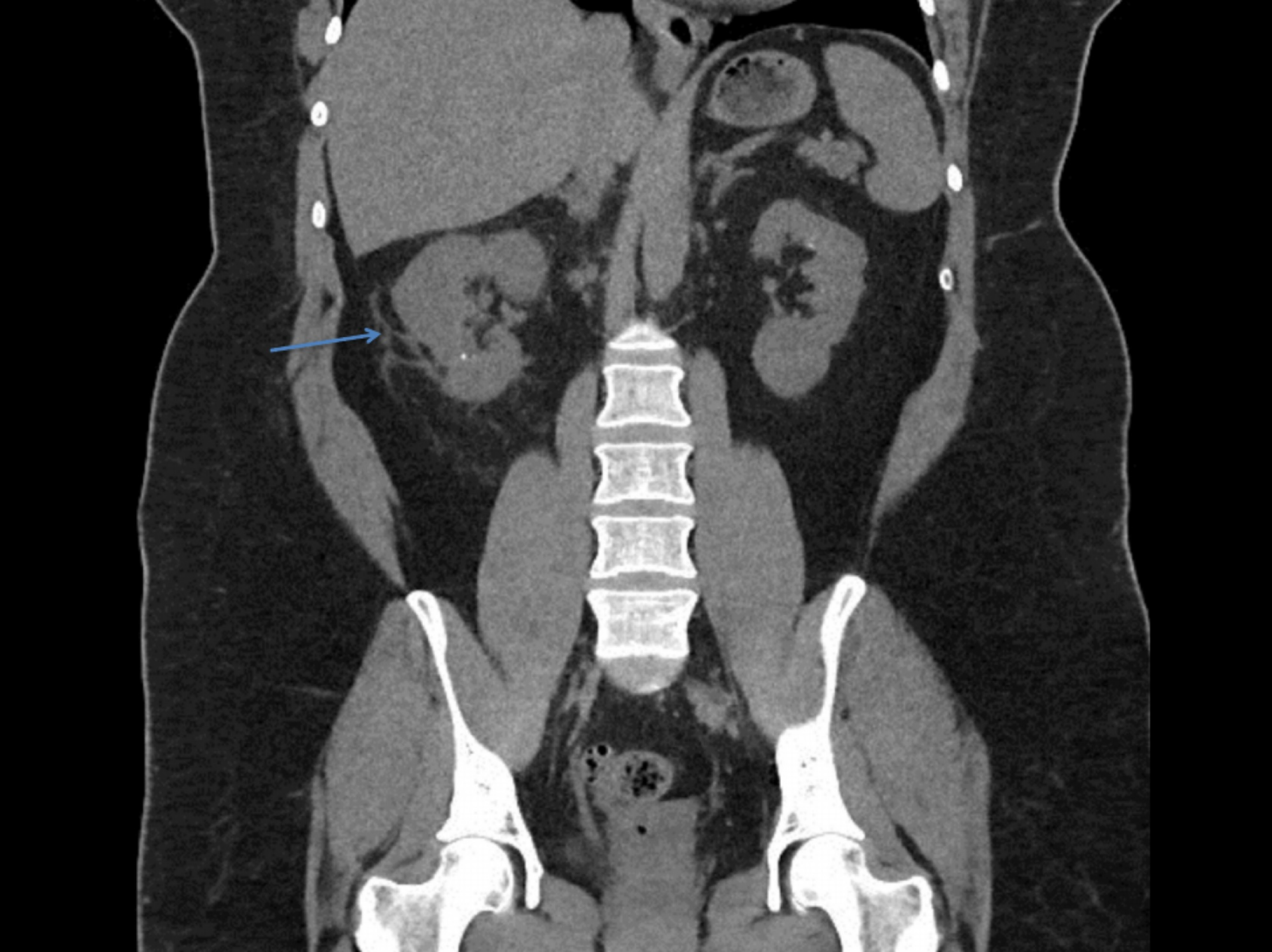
Computed tomography imaging of the abdomen and pelvis without contrast Computed tomography imaging of the abdomen and pelvis. Note the multiple small calculi in both kidneys with mild hydronephrosis, ureteral edema, and stranding of the right kidney and collecting system (arrow).
